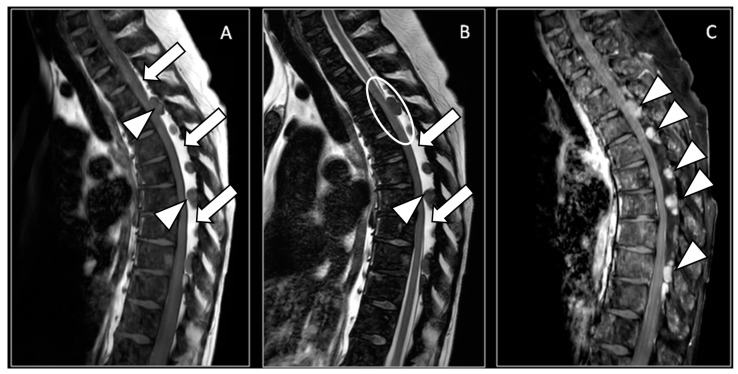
(A) Sagittal magnetic resonance T1-weighted image; (B) sagittal magnetic resonance T2-weighted image; (C) sagittal magnetic resonance T1 fat-suppressed weighted image after endovenous contrast media administration: a thick posterior epidural lipomatosis (white arrows), with high signal in A and B and low signal in C, that compresses the dural sac, in particular in the dorsal tract. The epidural lipomatosis should not be confused with the spinal cerebrospinal fluid, which demonstrates a high signal only in B and can be appreciated only in the cervical and the distal dorsal tracts. Within the lipomatosis, there are multiple solid nodulations, with a low signal in (A,B), characterized by a high signal in (C) (white arrowheads), due to pathological contrast enhancement. One nodulation adds further cord compression at the D3 level, where the spinal cord appears compressed with a high hyperintensity signal on T2w sequences, suggestive of spinal cord distress (white circle in (B)). Note also the diffuse pathological signal in all sequences of the vertebral bone marrow.
